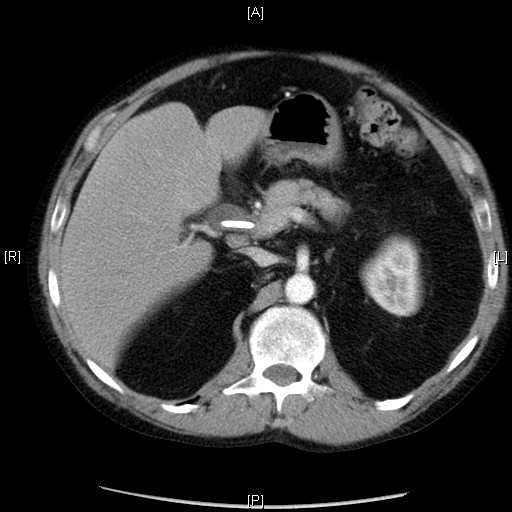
CT scan abdomen. Stent visible in bile duct.
